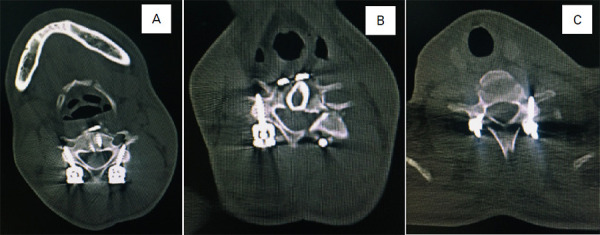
Postoperative computed tomographic axial section of C4 with lateral mass screws (A), C5 right lateral mass screw, fracture of left lamina and pedicle of C5, and autologous fibular strut graft at C5 corpectomy site (B), C7 left lateral mass screw, and right pedicle screw (C).
